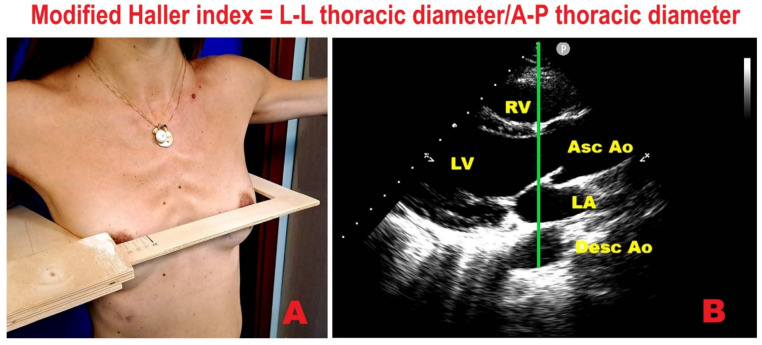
Modified Haller Index. (A) Latero-lateral external thoracic diameter measured with a rigid ruler and level at the distal third of the sternum. (B) Antero-posterior internal thoracic diameter obtained on transthoracic echocardiography in the parasternal long-axis view, measured as the distance from the true apex of the ultrasound sector to the posterior wall of the descending aorta immediately behind the left atrium. Ao, aorta; Asc, ascending; Desc, descending; LA, left atrium; LV, left ventricle; RV, right ventricle.
